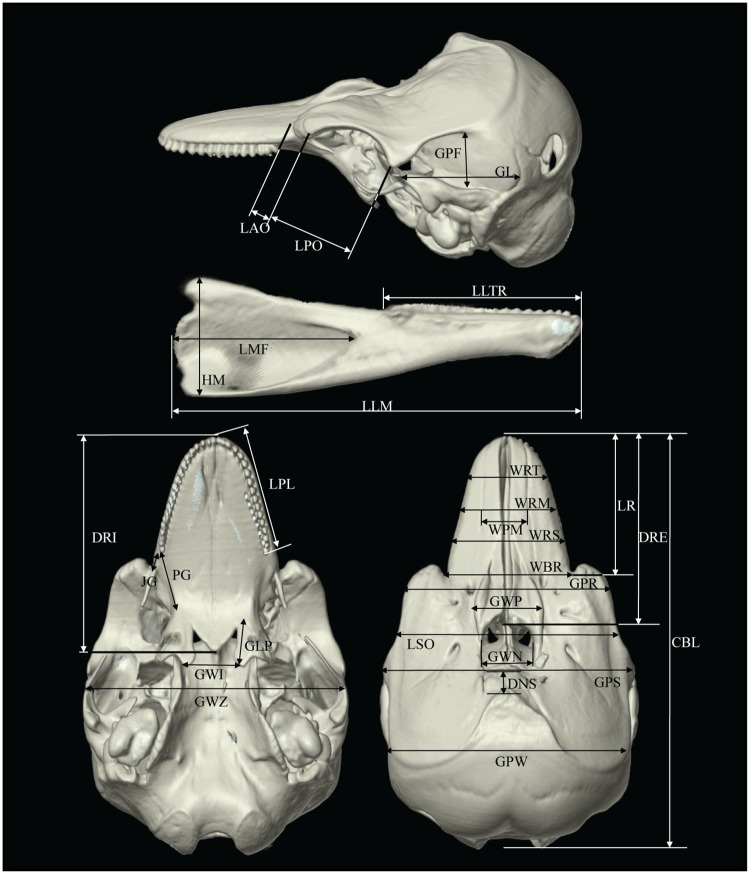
Key to cranial measurements made on CT 3DVRI of *Neophocaena phocaenoides*. For abbreviations, refer to Table 1.
